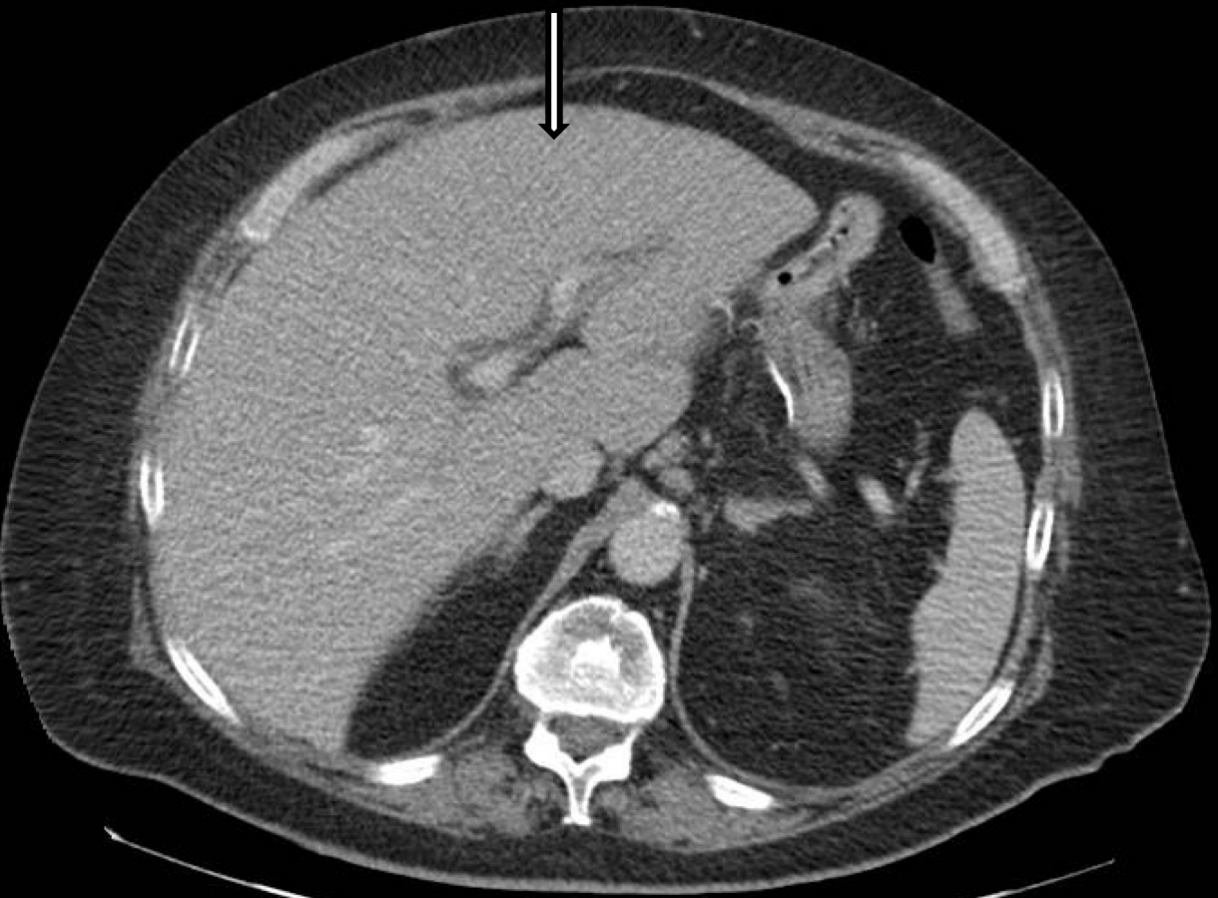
Computed Tomography (CT) of the Abdomen with Intravenous contrast Axial image at the level of the liver demonstrates a mildly enlarged liver without a discrete focal mass (arrow)
